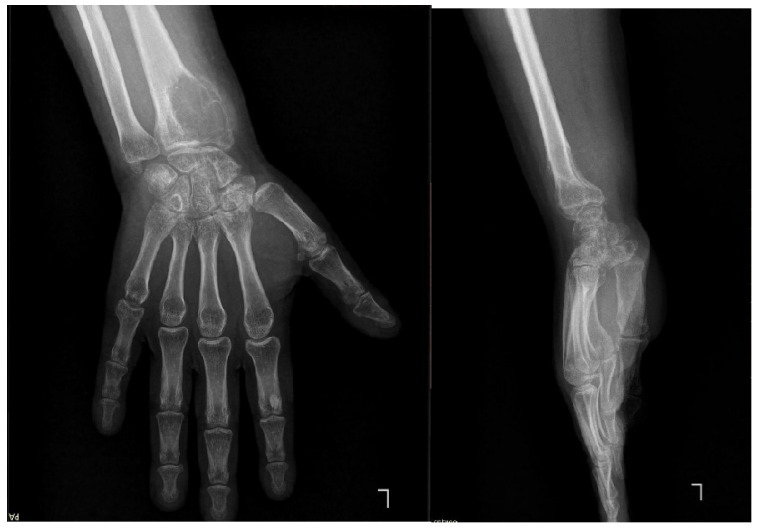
Anteroposterior and lateral wrist radiographs demonstrating an expansile osteolytic lesion at the level of the distal epiphysis of the left radius, suggestive of a giant cell tumor (GCT).
